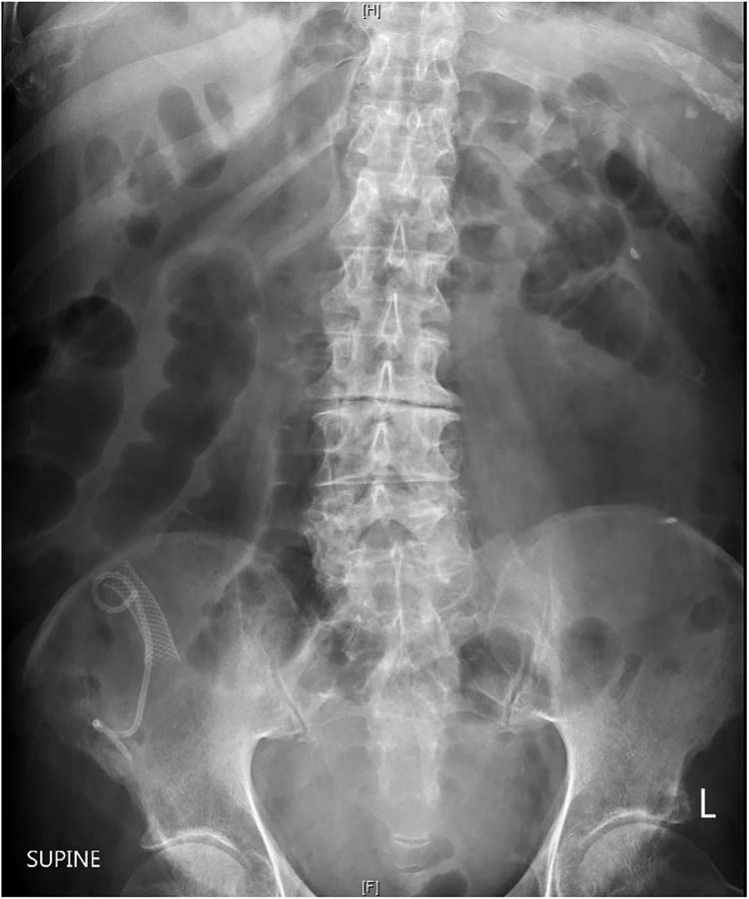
Abdominal radiography showing plastic and metallic biliary stents projected over the right iliac bone and dilated loops of small bowl throughout the abdomen and pelvis.
